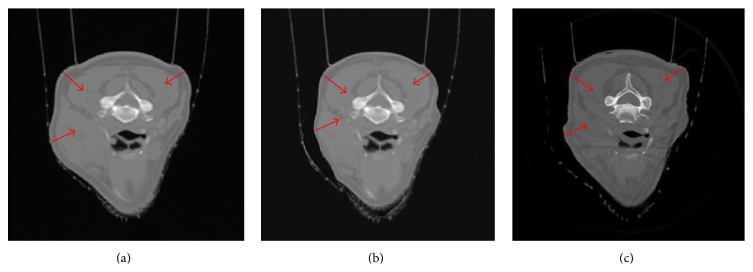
Demonstration of H&N01 patient results from axial view. (a) Original CT image; (b) deformed image; (c) target image (daily CBCT).
